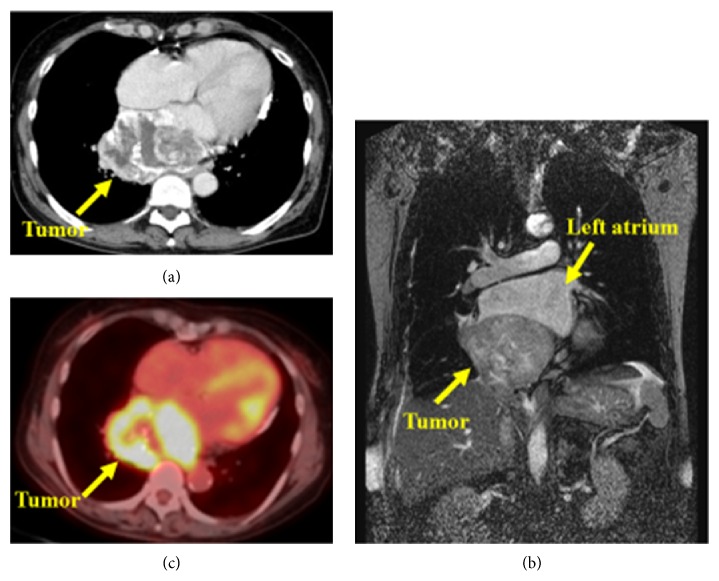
(a) Dynamic CT shows the mass 65 × 90 mm in diameter in the middle mediastinum which is hyperdynamic enhancement continuing from the early to delayed phase. (b) MRI of the chest demonstrating a large tumor compressing the left atrium. The tumor and left atrium are indicated with arrows. (c) PET-CT image showing a mediastinum mass with the SUVmax of 10.5.
